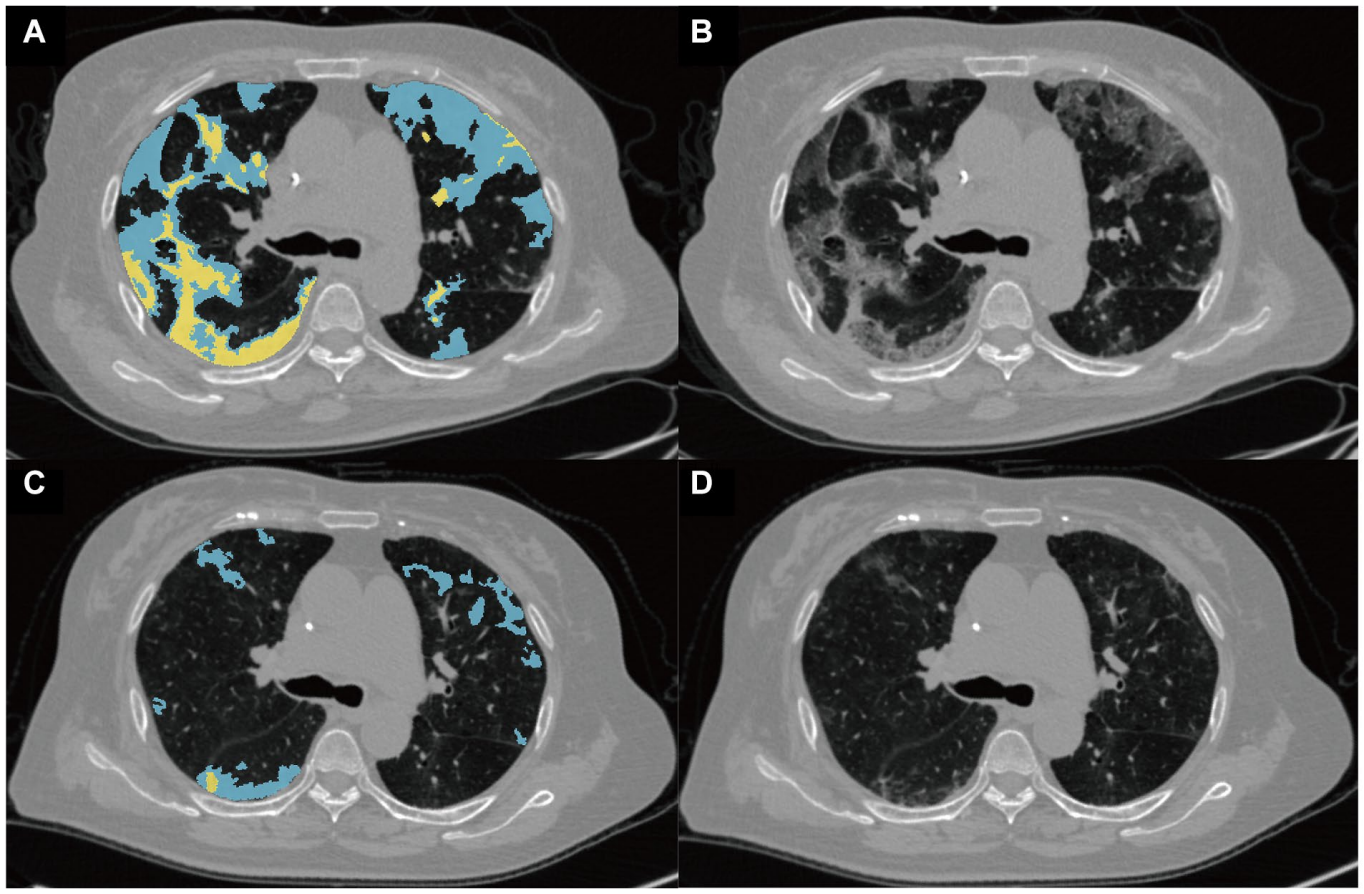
Representative images from the quantitative analysis of pneumonia lesions. Pneumonia lesions at the baseline (A) and follow-up (B) CT scans of a 75-year-old woman with critical COVID-19 were segmented into ground-glass opacity (GGO) and consolidation, shown in blue and yellow, respectively. The raw baseline and follow-up images of this patient are shown in (C,D), respectively.
